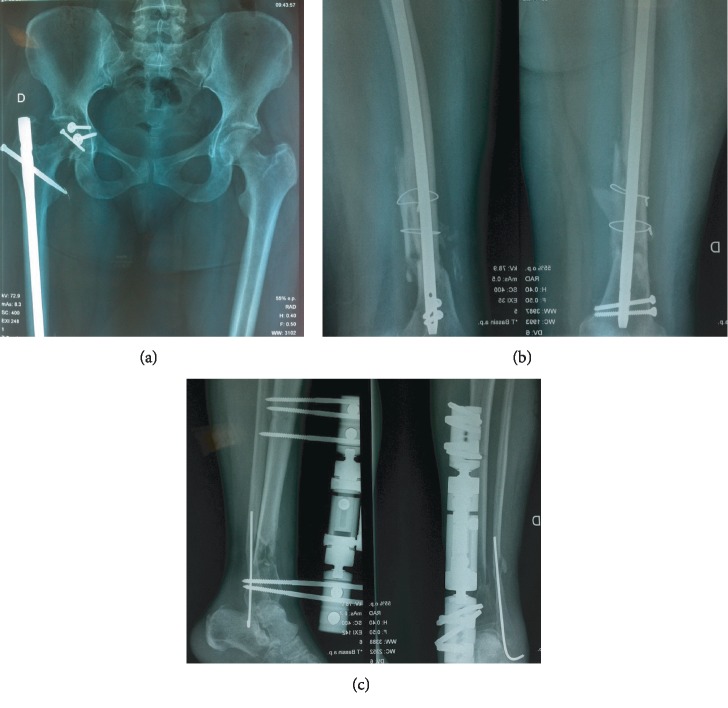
Radiographs of the (a) pelvis, (b) femur, and (c) tibia at 7-month follow-up.
